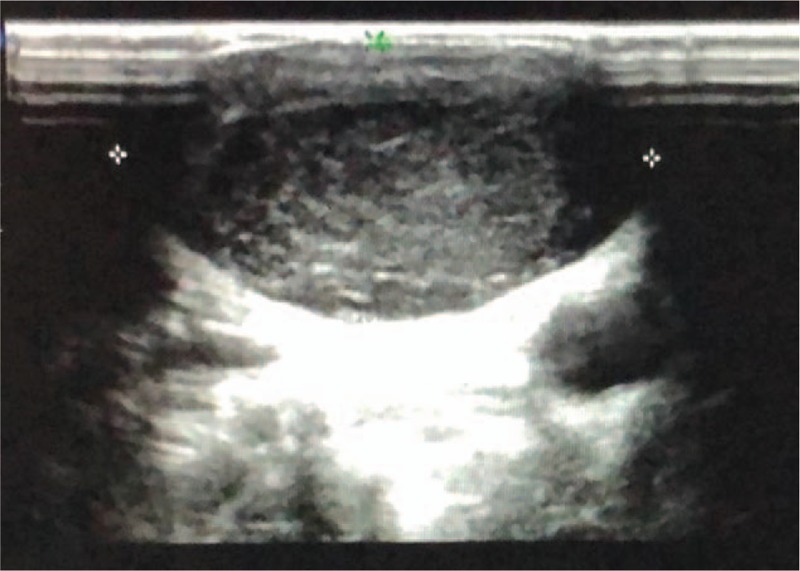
Ultrasound examination revealed a well-circumscribed hypoechogenic image of the right neck.
